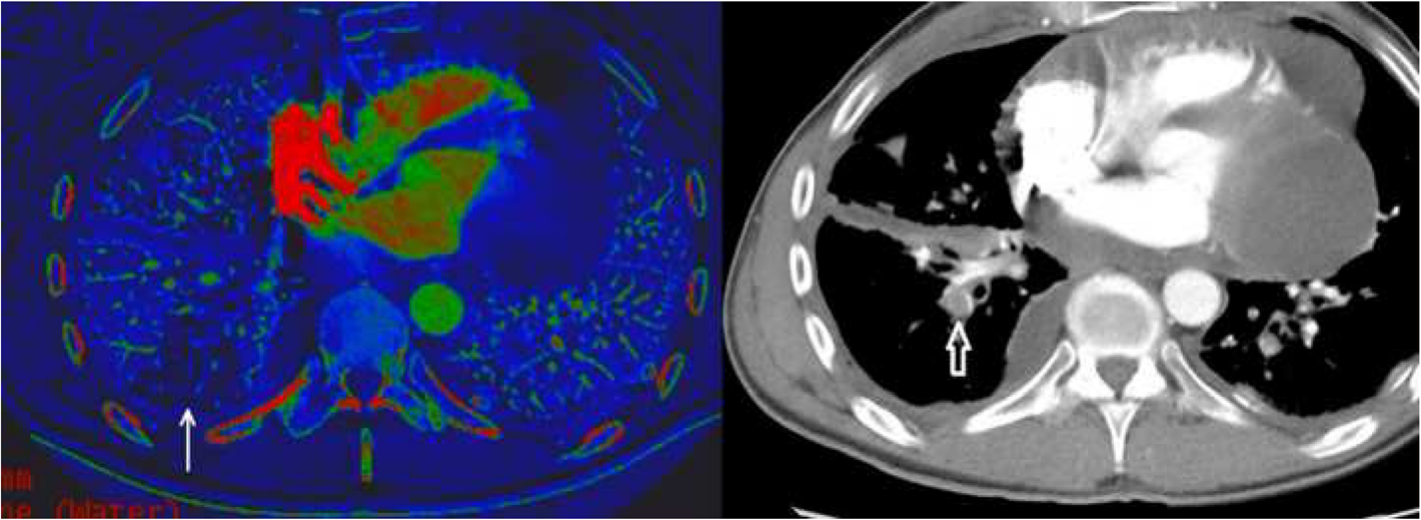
Dual energy CT in a patient with pericardial and pleural metastases, with a PE. Iodine map is shown with perfusion defect (white arrow). This is correlated to the region with the PE in the right lower lobe (open white arrow)
